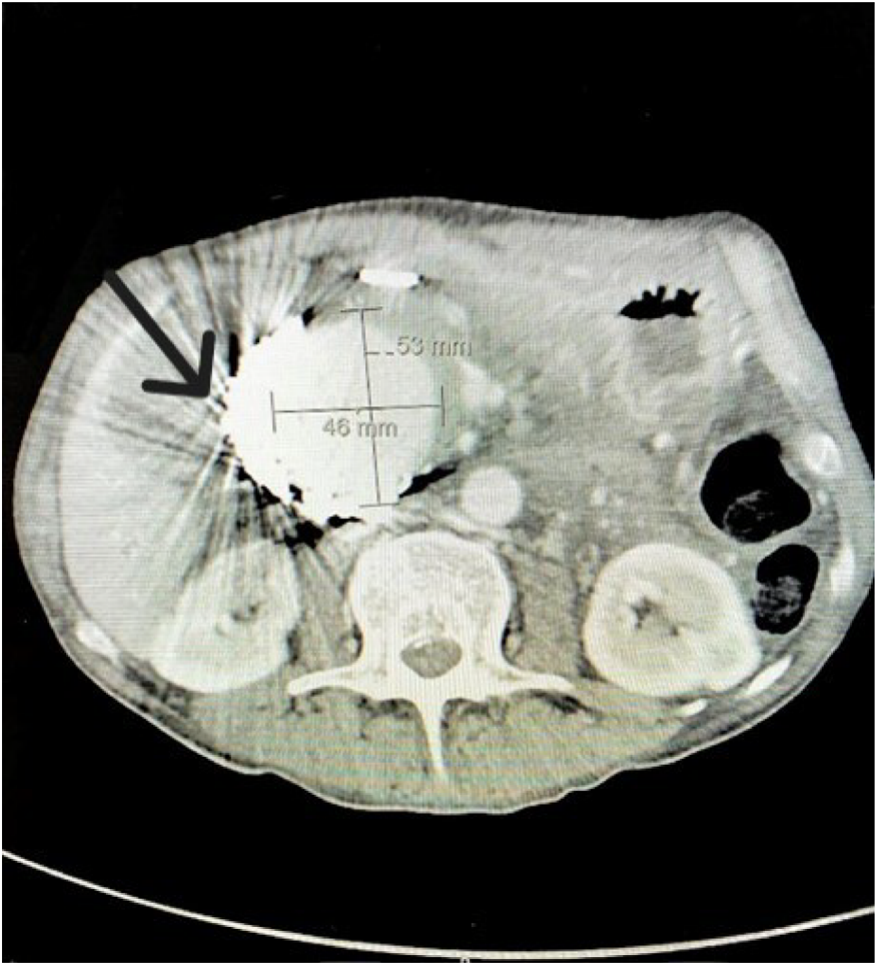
Axial contrast-enhanced computed tomography in the arterial phase, pre-procedure, demonstrating a large visceral pseudoaneurysm in the right upper quadrant (brackets with measurements); and an artifact from the endovascular coils within the lesion from prior embolization (arrow).
